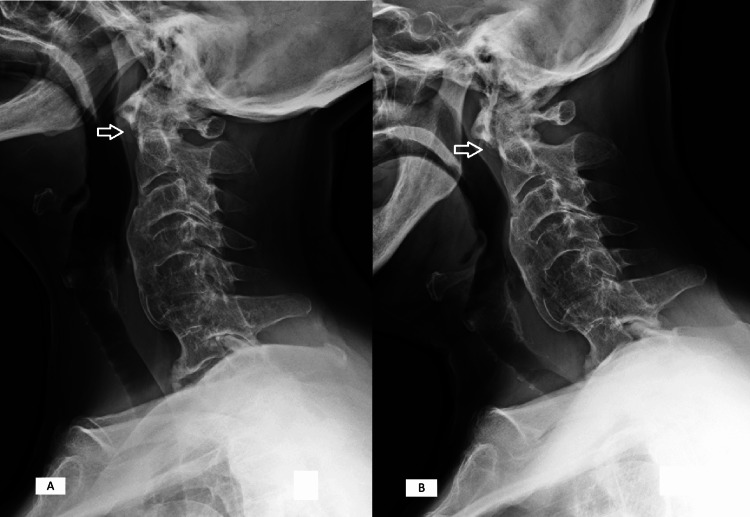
Dynamic X-rays (A) Hyperextension; (B) hyperflexion. Arrow shows slight C1-C2 subluxation, with an increase of the anterior atlanto-odontoid interval between images A and B.
